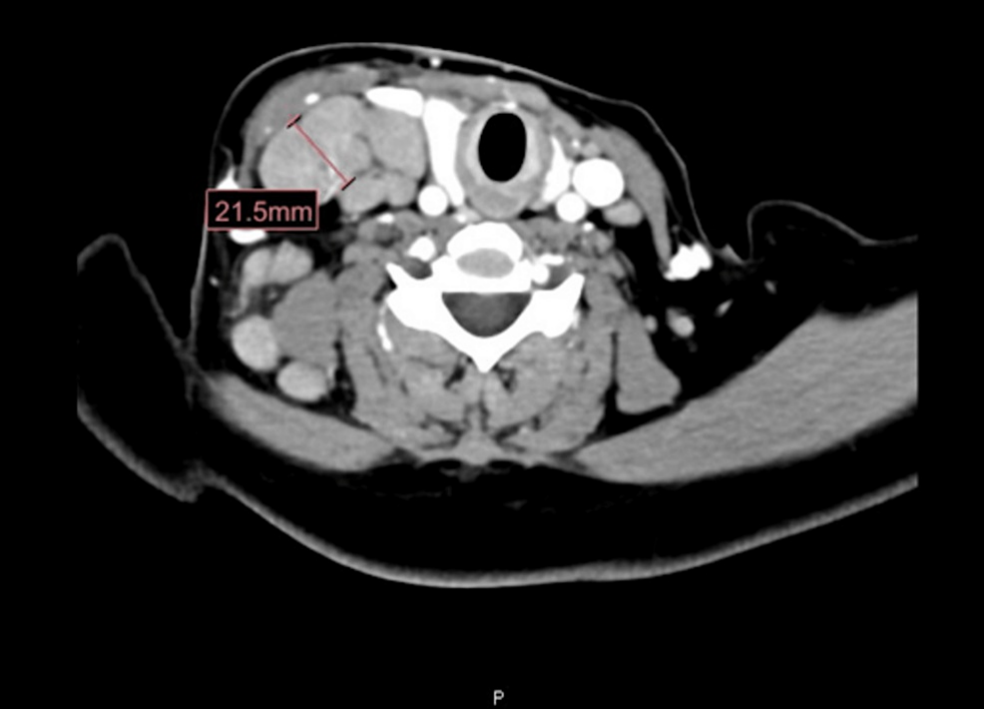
CT neck with contrast (axial view) showing severe bilateral neck lymphadenopathy most prominent on the right side at the jugulodigastric region level (2A and 2B), uplifting the right sternocleidomastoid muscle CT, computerized tomography
